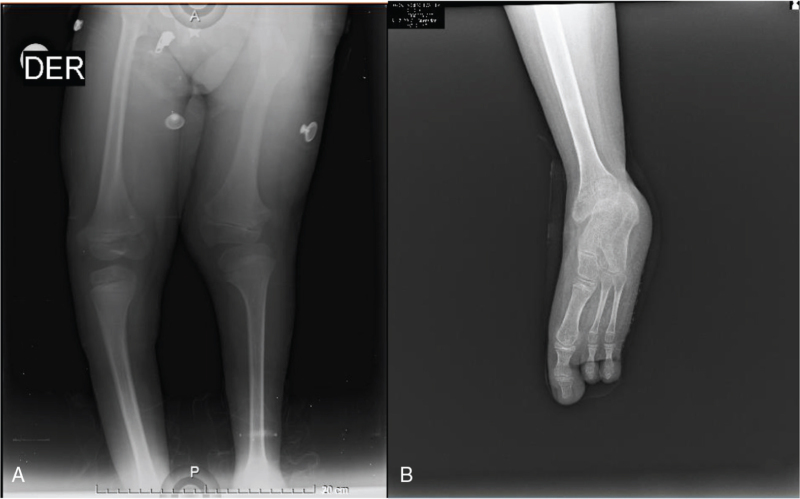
(A) Anteroposterior femur, tibia X ray, bilateral fibular hemimelia with left short femur. (B) Oblique left foot radiograph demonstrates deficiency of the fourth and fifth ray, only 1 cuneiform can be observed and tarsal coalition.
